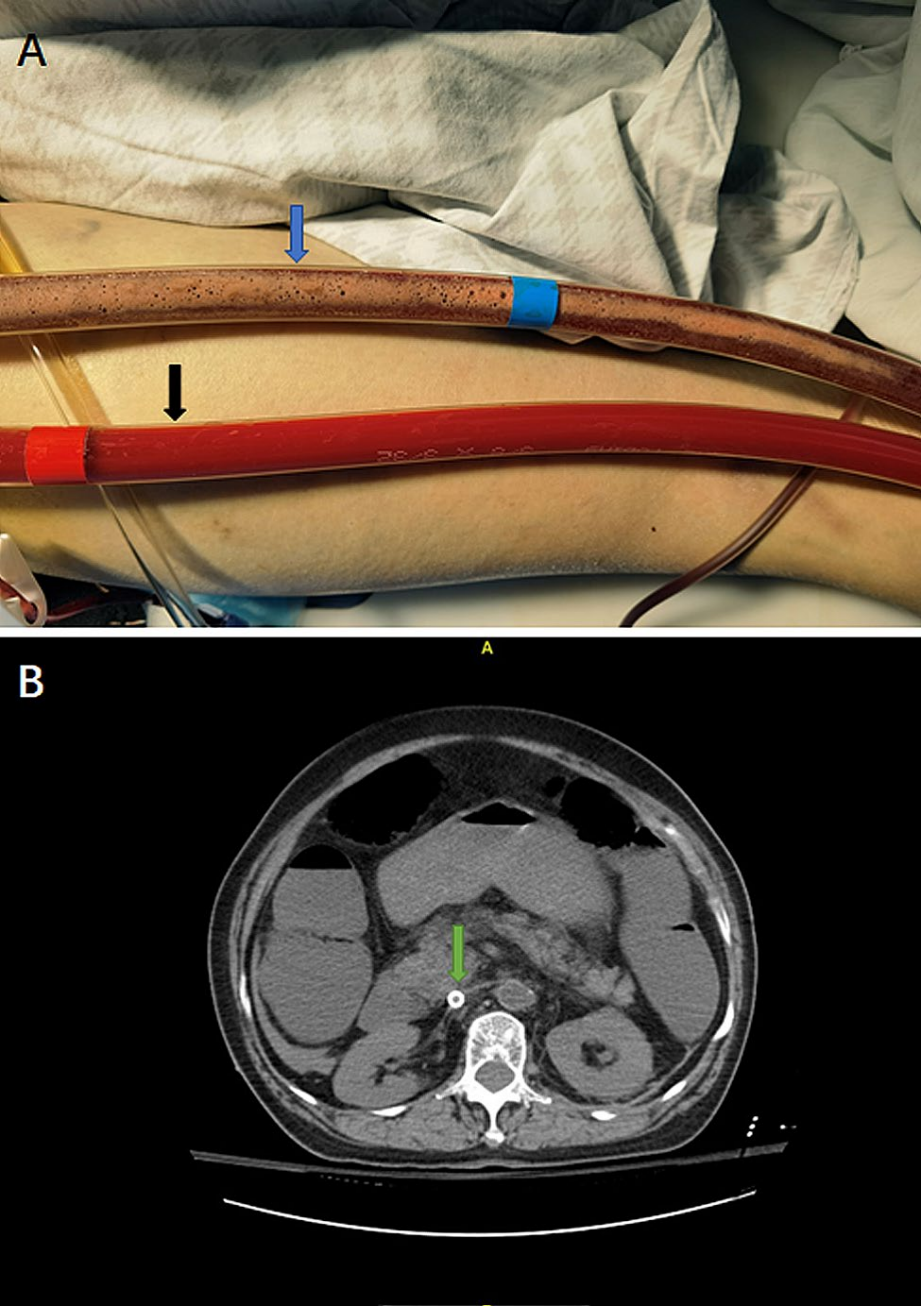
(A)The bright red tube is the arterial line of the ECMO (black arrow); the dark tube with many bubbles is the venous line of the ECMO (blue arrow). There are many small bubbles were formed and attached to the venous line of the ECMO. (B) Abdominal computed tomography showed that the inferior vena cava had collapsed completely(green arrow)
